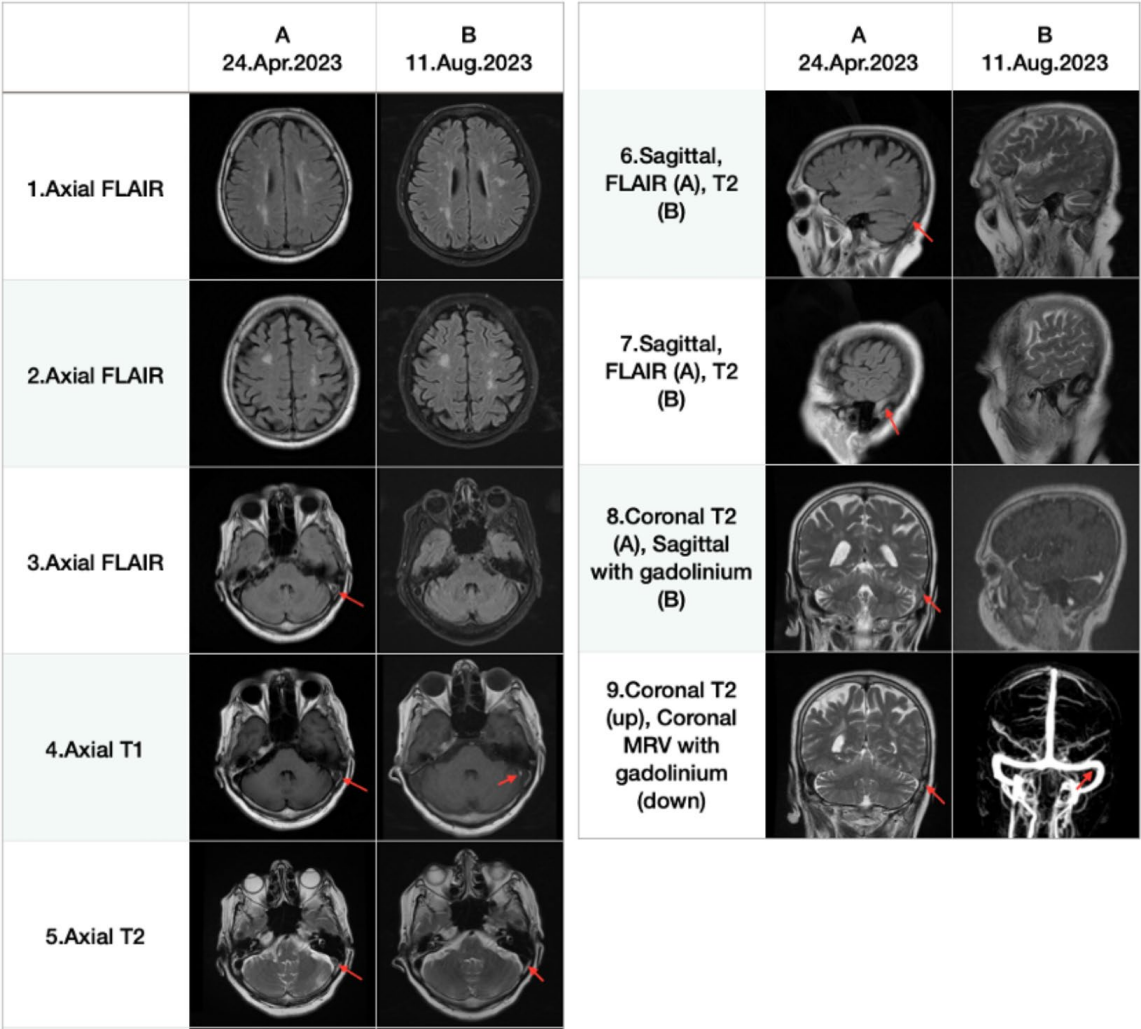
Comparison between the two MR images of the patient taken 7 months apart. In the axial flair images, subcortical white matter lesions were identified in images A1, A2, B1, and B2. A filling defect was observed in the left transverse sinus and sigmoid sinus, spanning images A3–A7, as indicated by a red arrow. Notably, images B3–B7 depict an almost complete resolution of the previously noted defects in the left transverse sinus and sigmoid sinus, with a residual small filling defect persisting in image B4. Additionally, a small filling defect was present in images B8 and B9, with the latter distinctly marked by a red arrow.
